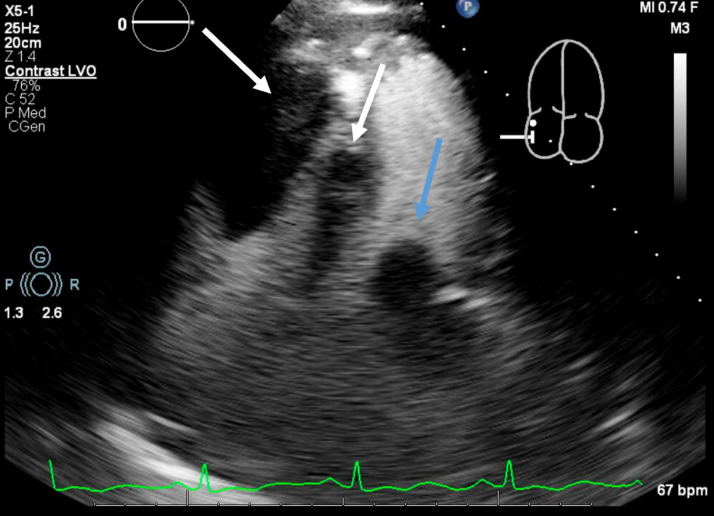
Transthoracic Echocardiography at Day 20 With Contrast Echo Demonstrating Right Ventricular Masses and a Left Atrial Mass The white arrows point to the right ventricular masses, and the blue arrow points to the left atrial mass.
